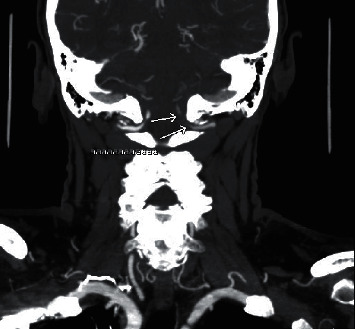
CTA showing left vertebral artery dissection in coronal plane.
